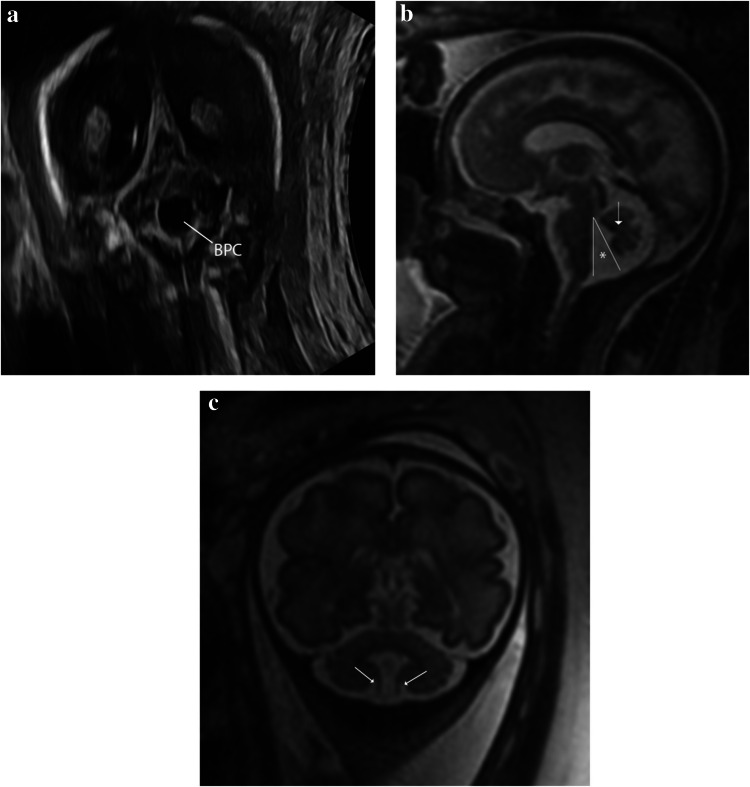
Persistent Blake pouch cyst versus vermian hypoplasia. a Prenatal ultrasound of the fetal head in the coronal plane at 20 6/7 weeks shows an anechoic space between the two cerebellar hemispheres, reflecting the CSF-filled Blake pouch cyst (BPC). b, c A different case of suspected BPC versus mild vermian hypoplasia is shown by fetal MRI at 30 3/7 weeks, with upward rotation of the vermis and enlarged tegmento-vermian angle (*asterisk*) evident on the midline sagittal SSFSE view (b). Note also the sharp fastigial recess (*arrow*), helping to distinguish BPC from a Dandy-Walker phenotype. Although a normal shape of the vermis is maintained, the primary fissure is prominent, and the subjective volume of the inferior vermis is slightly less than expected, warranting consideration of inferior vermian hypoplasia. However, postnatal brain MRI at term showed the normal appearance of the vermis and a normal, narrow tegmento-vermian angle (not shown). Coronal SSFSE (c) shows the medial position of the taenia-tela choroidea complex (*white arrows*), as seen in the presence of BPC
